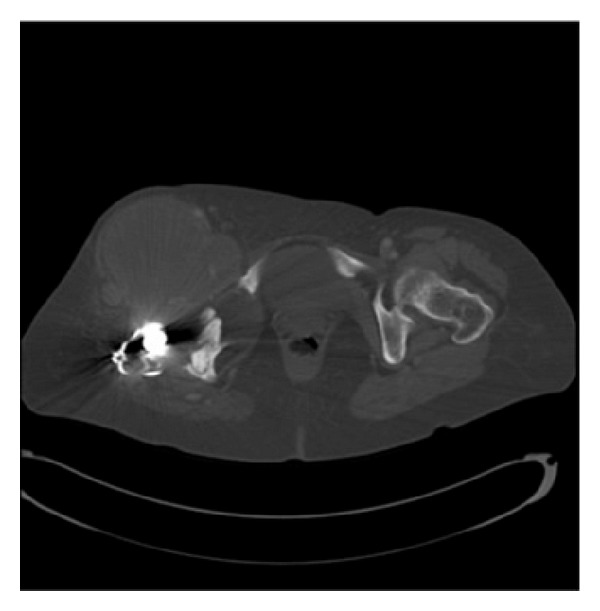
CT scan showing the mass originating from the right hip joint and extending predominantly anteriorly and medially.
